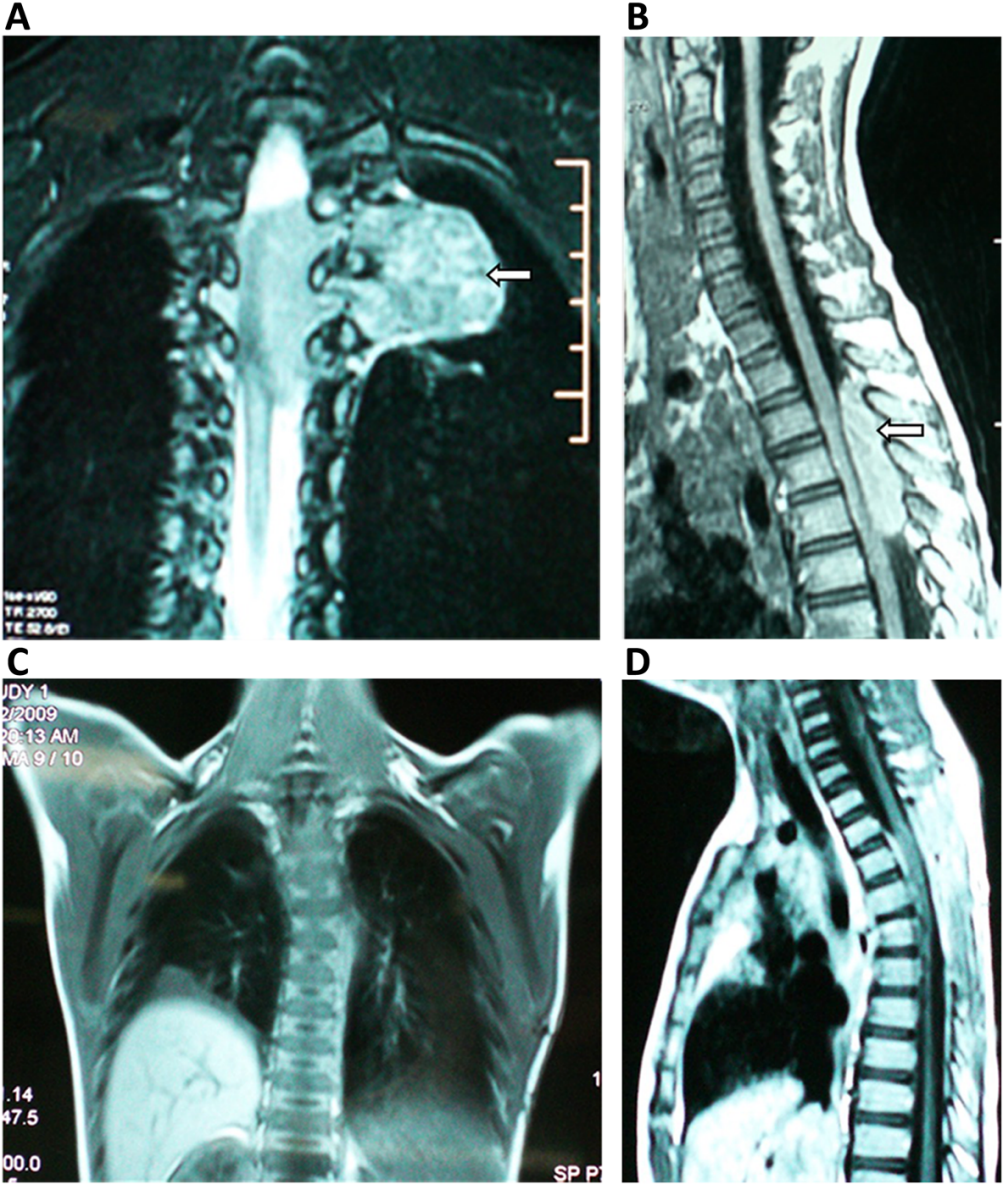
Magnetic resonance imaging (MRI) before (a, b) and after treatment (c, d). a the chest coronal MRI showed a dumbbell-shaped mass (arrow) extending to the spinal canal and paravertebrally through an enlarged intervertebral foramen; b sagittal MRI indicated that the mass was located in the spinal canal between T3 and T5 (arrow) and measured up to 5 cm in length. The spinal cord was compressed and displaced; c coronal MRI showed that there was no mass found in the thoracic cavity; d sagittal MRI showed that there was no mass found in the spinal canal between T3 and T5
